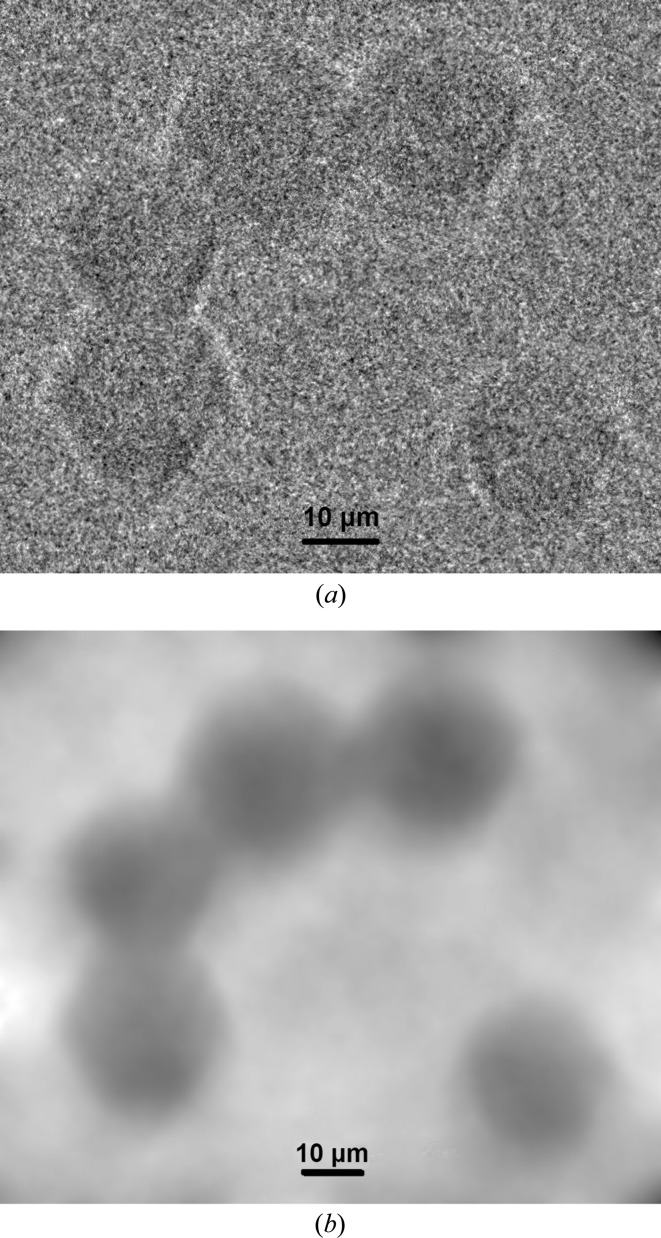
(*a*) Single X-ray projection of PMMA spheres (diameter of 20 µm) after flat-field correction and (*b*) the retrieved phase image (relative electron density) of PMMA spheres.
